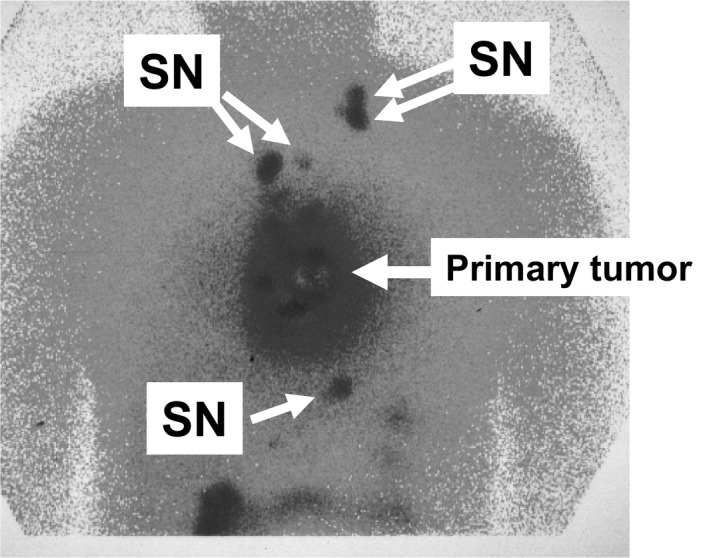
Preoperative lymphoscintigraphy in thoracic esophageal cancer. SN, sentinel node
